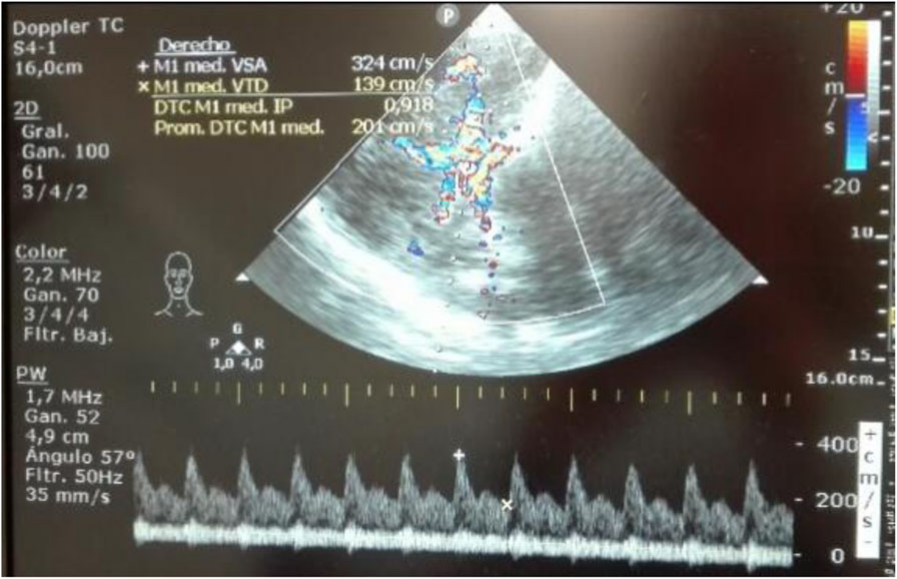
Circle of Willis and Pulsed-wave Doppler mode of middle cerebral artery
